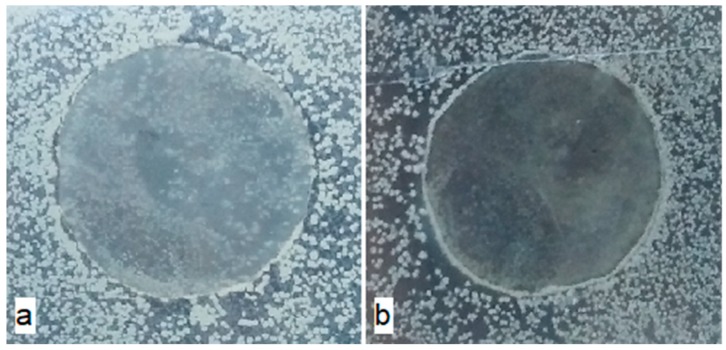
External appearance of polyethylene without birch bark extract (a) and polyethylene with 1.0% content of birch bark extract (b) and the use of ultrasonic treatment after the incubation under the influence of *C. albicans* for 24 h.
